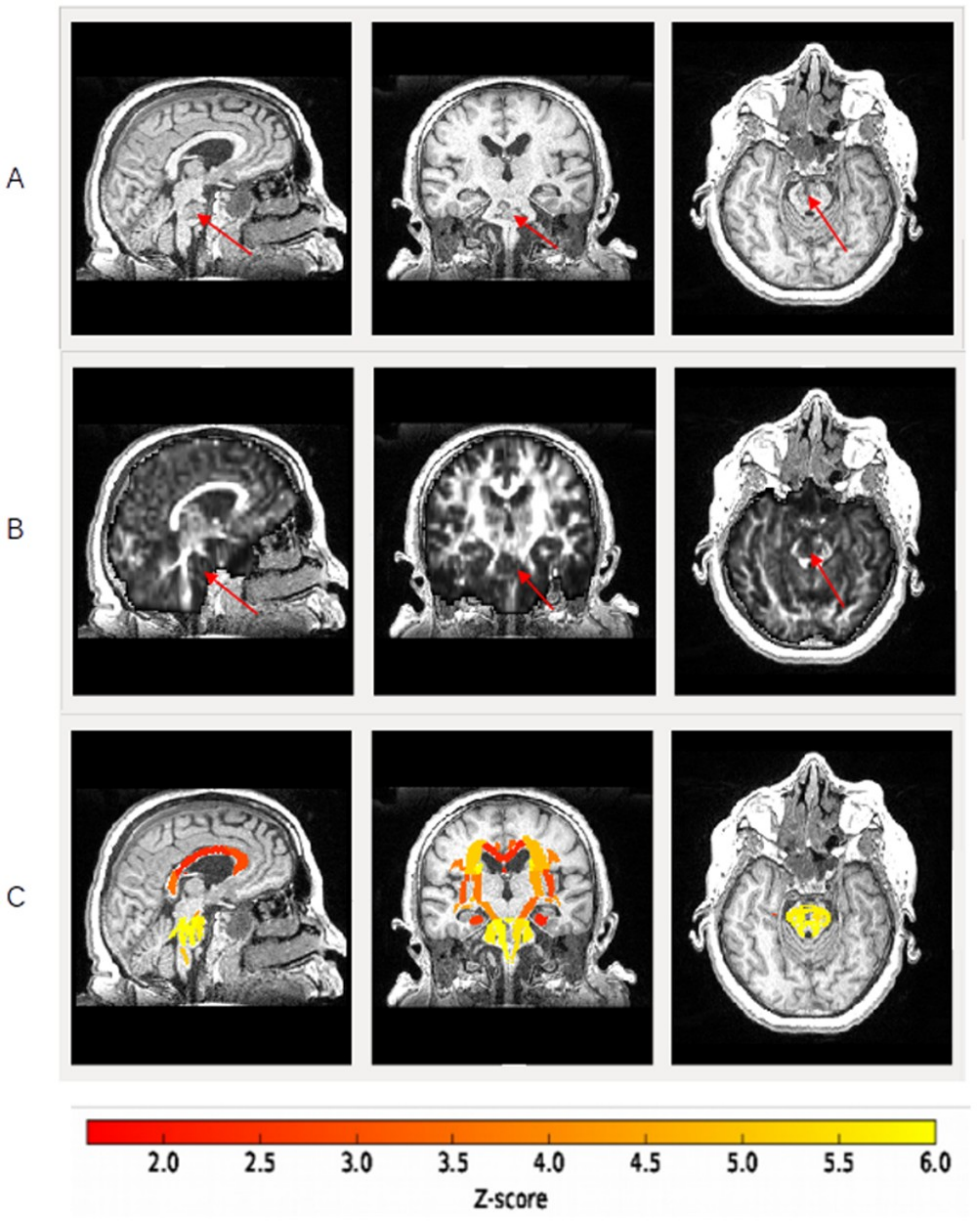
Z-score results. A: T1-weighted images of a LIS patient (sagittal, coronal and axial slices). Arrows indicate the lesion in the pons. B: FA images (sagittal, coronal and axial slices) of a LIS patient. Arrows indicate the lesion in the pons. C: Example of FA Z-score statistic (absolute values) on T1-weighted images (sagittal, coronal and axial slices) for a LIS patient and for the 48 white matter fiber tracts studied: yellow indicates the highest Z-scores and red the lowest Z-scores (see colour bar just under the image). The patient shows a severe injury at the pons level.
